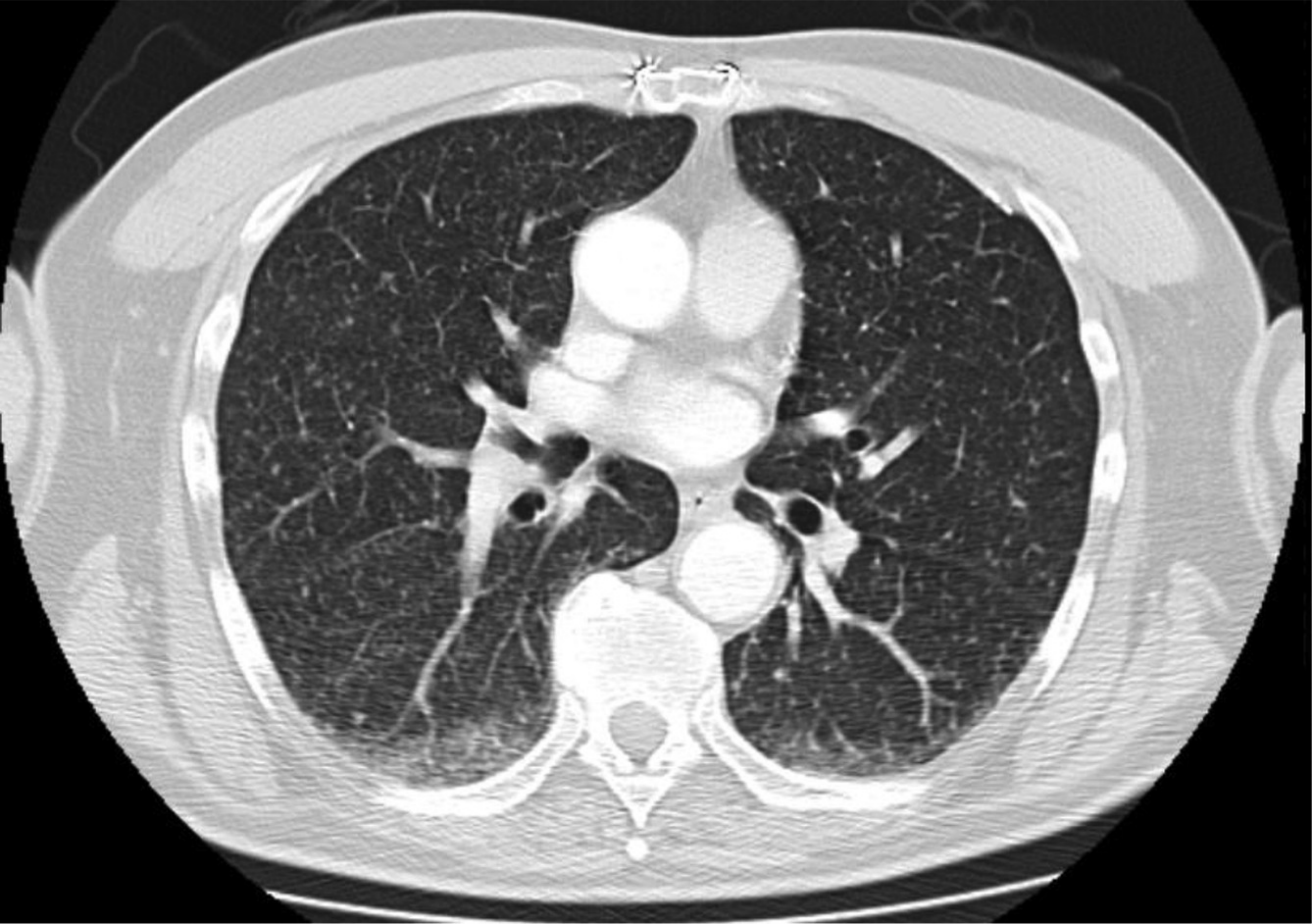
Computed tomography scan of chest with reported miliary changes.
